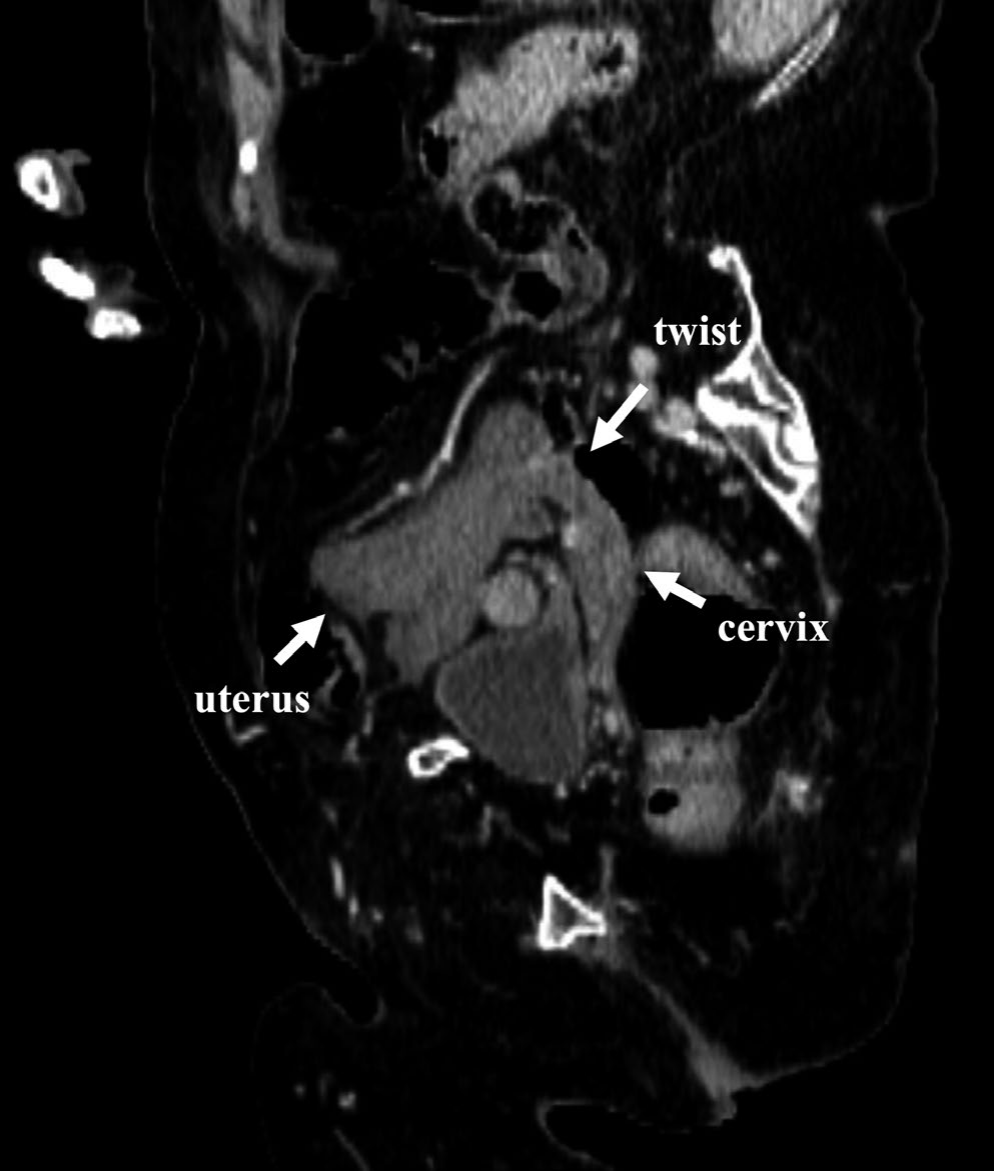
CT of the abdomen and pelvis shows torsion of the cervix (arrow)
